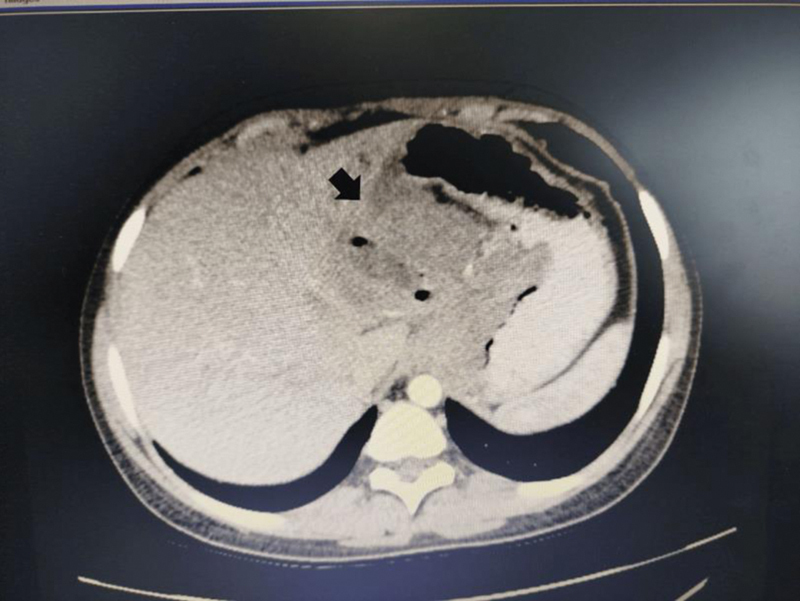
Axial CT scan showing multiple hypodense liver lesions (
